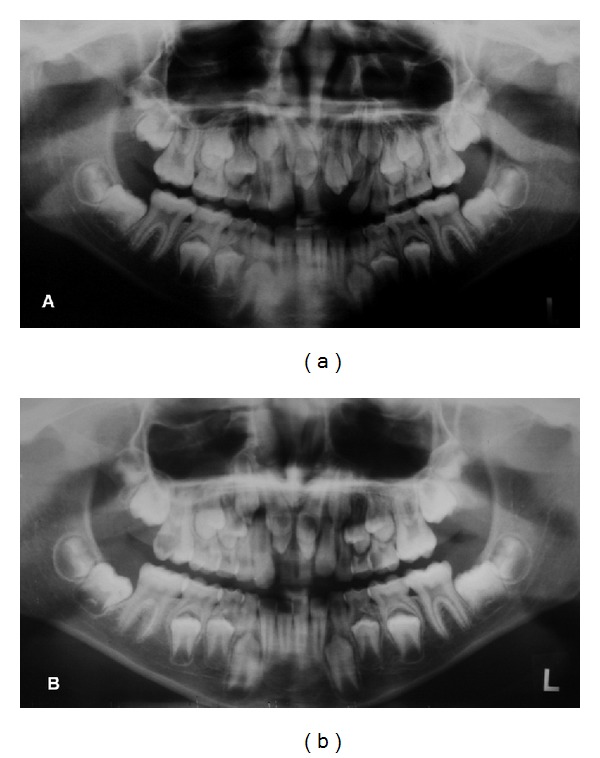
Panoramic radiograph showing impacted permanent maxillary central incisors and the presence of two mesiodens in both twins.
